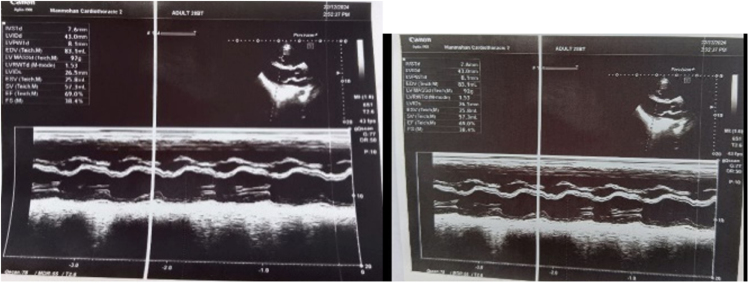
Echocardiographic finding shows hypoechoic oscillating structure of 32 mm × 9 mm attached to the pulmonary valve, with dilated right atrium and right ventricle.
